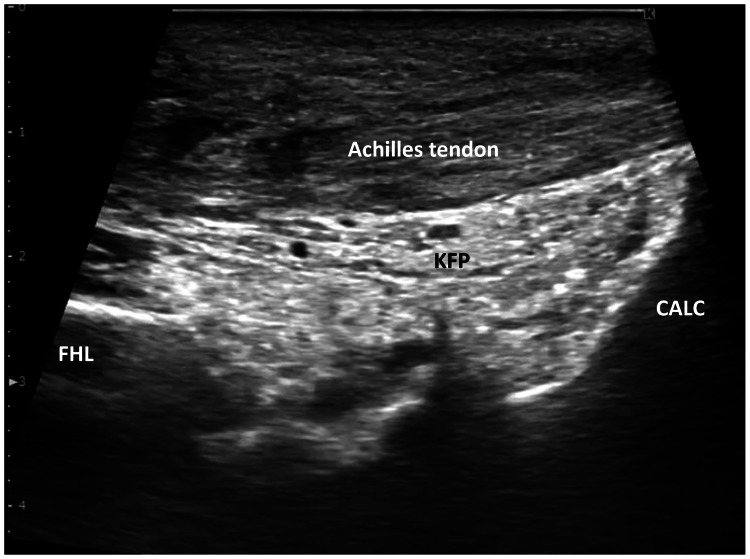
Static ultrasonography of the Achilles tendon before ultrasound-guided manual therapy (8-weeks post-surgery) CALC: calcaneus; FHL: flexor hallucis longus muscle; KFP: Kager’s fat pad.
